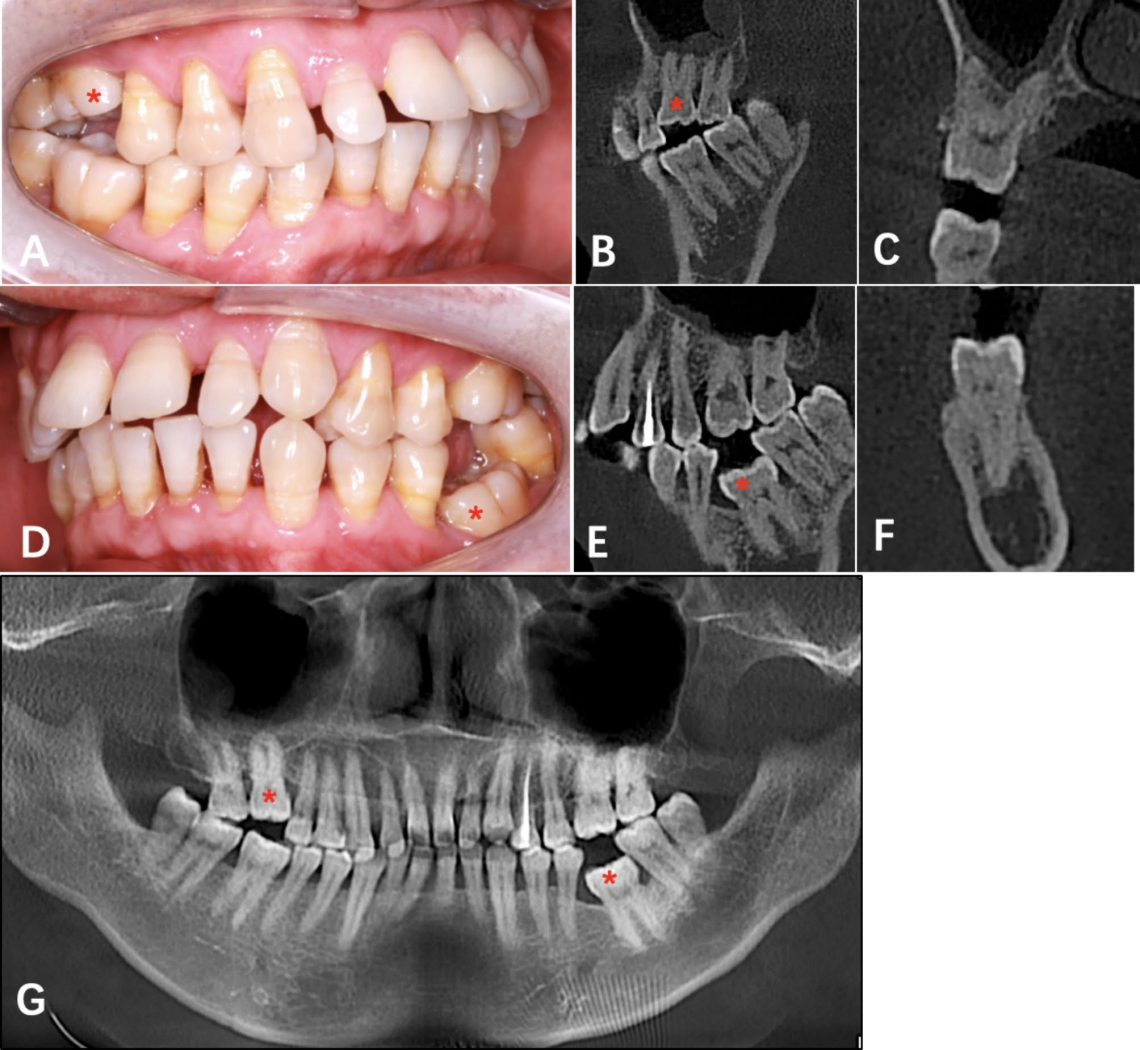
Clinical and radiographic information of the proband’ father. (A, intraoral right view. B, the sagittal image of 16. C, the coronal image of 16. D, intraoral left view. E, the sagittal image of #36. F, the coronal image of #36. G, panoramic radiograph. “*” represent the PFE involved tooth.)
